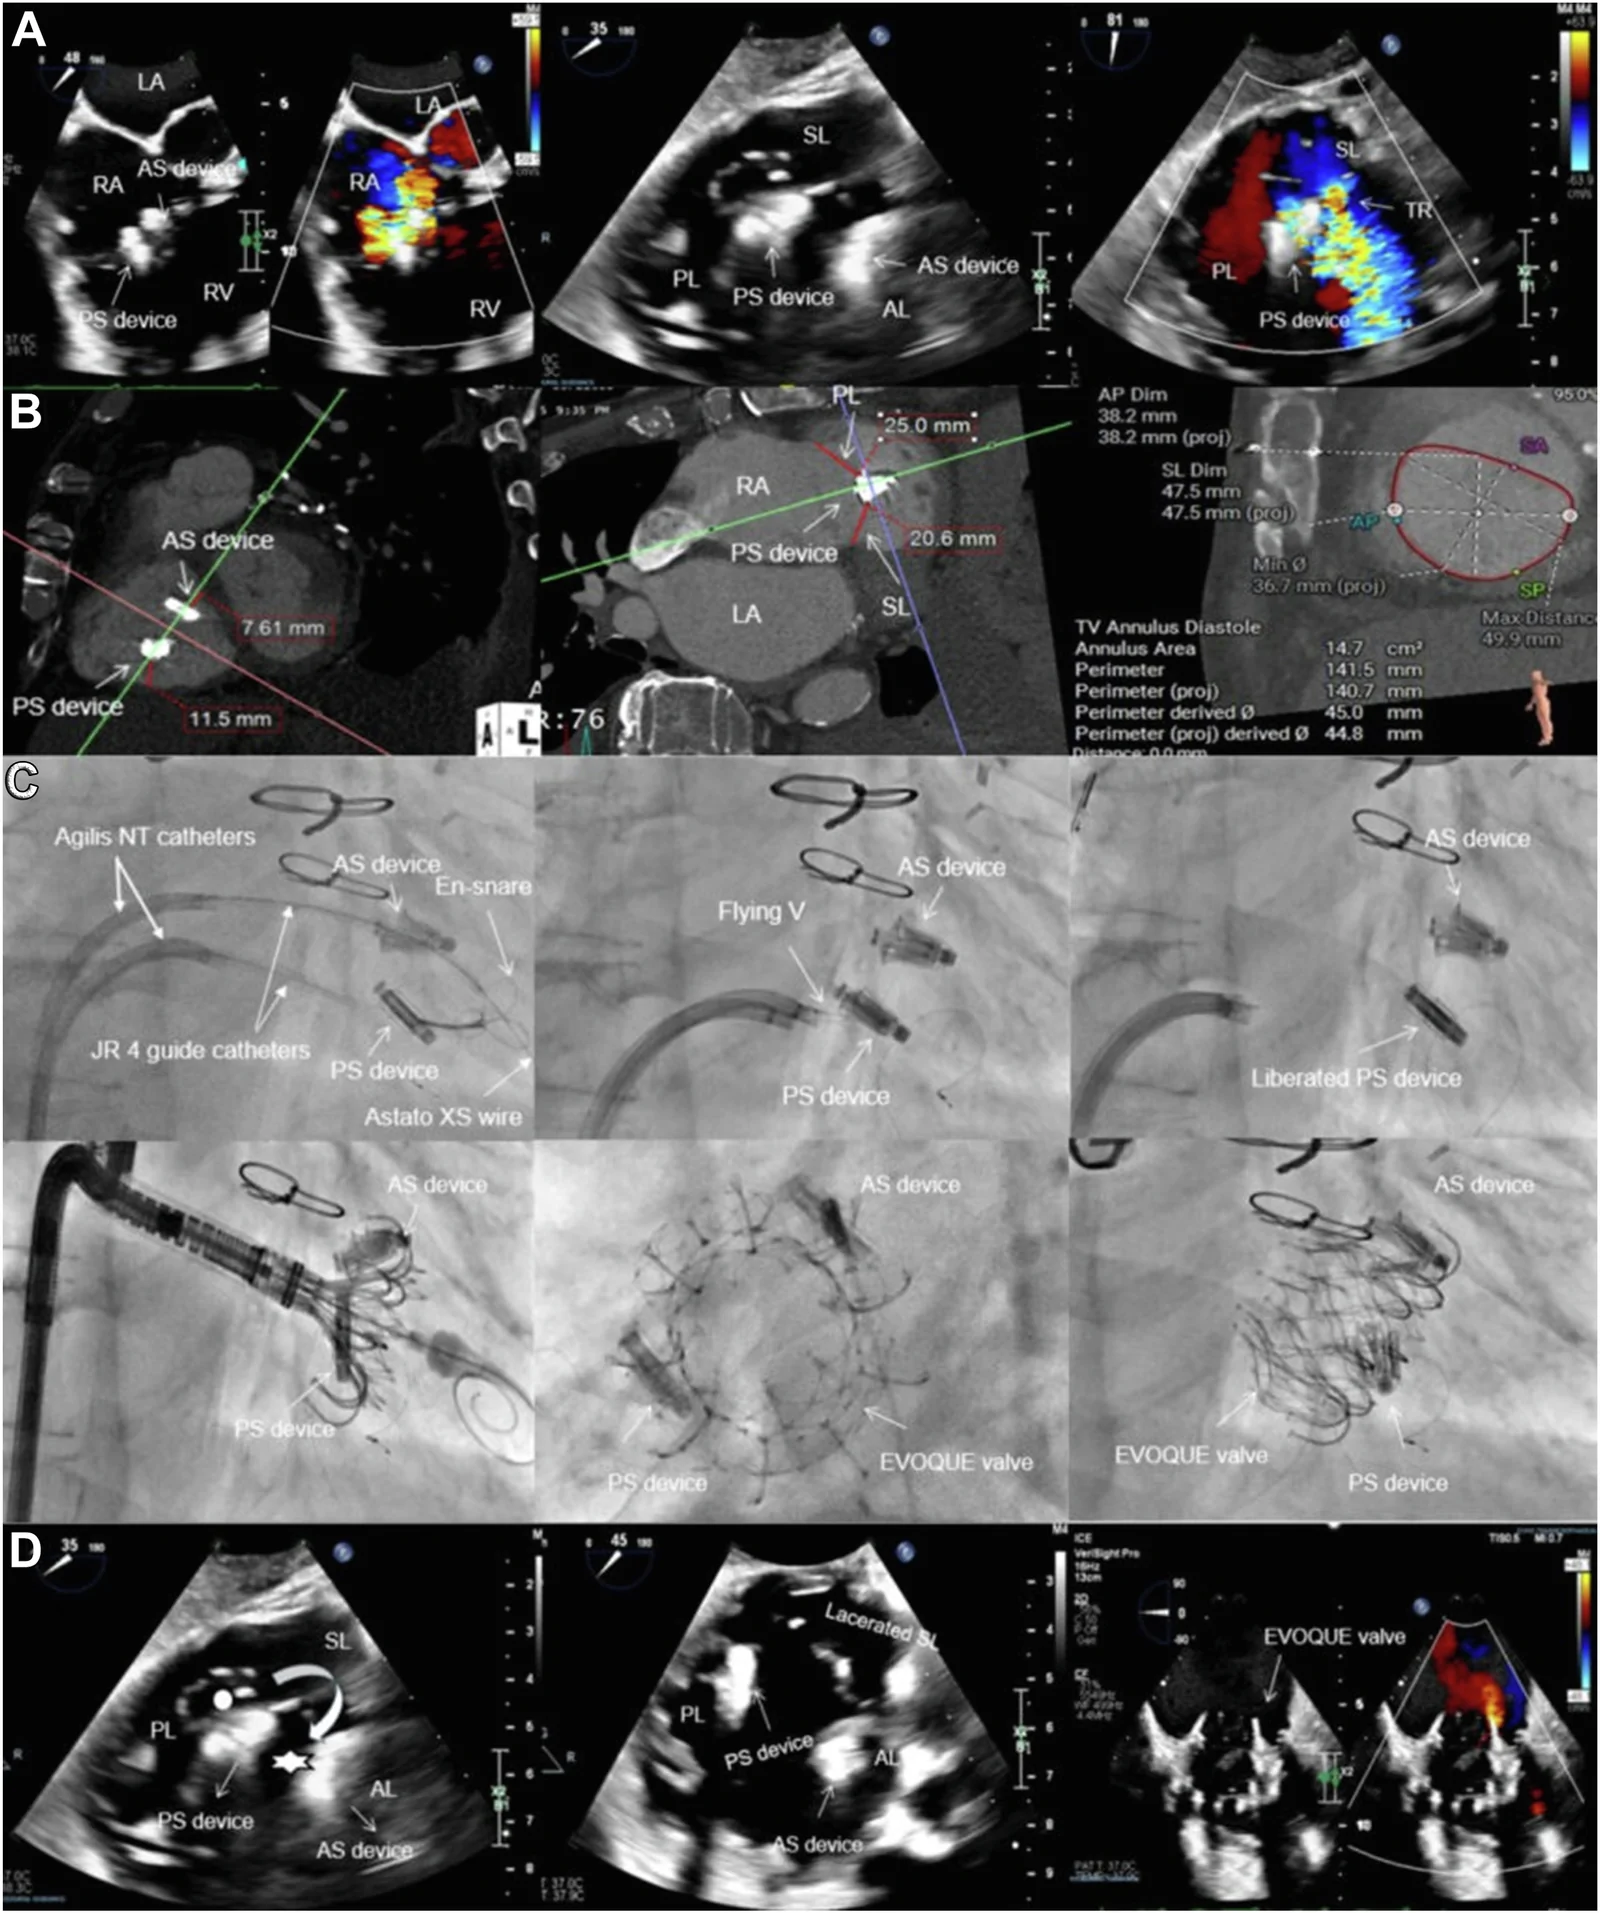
Multimodality imaging of tricuspid TEER device laceration and TTVR. (A) Transesophageal echocardiography demonstrating tricuspid TEER devices and severe TR. Mid-esophageal right ventricular (RV) inflow–outflow view showing anteroseptal (AS) and posteroseptal (PS) TEER devices with color Doppler demonstrating recurrent severe TR. Transgastric short-axis view showing PS device with poor septal leaflet tissue bridge. Severe TR from septal–posterior coaptation gap. (B) Cardiac CT showing device-to-annulus distance, leaflet length, and neo-annular dimensions for TTVR sizing. (C) Fluoroscopy of PS device laceration and TTVR. Astato wire snared with an EN Snare across the PS device. Flying-V configuration around the septal aspect of the PS device. Increased PS device mobility postlaceration. Safari wire in RV and EVOQUE valve deployment. EVOQUE anchors stabilize AS/PS devices. (D) Key transesophageal and intracardiac echocardiographic images of TEER device laceration and TTVR. TEE image showing the planned trajectory of electrocautery laceration (arrow), with the planned orifice for crossing (round and star). Septal leaflet-free PS device. ICE depicting EVOQUE valve with trivial residual TR. ICE, intracardiac echocardiography; TEE, transesophageal echocardiography; TEER, transcatheter edge-to-edge repair; TR, tricuspid regurgitation; TTVR, transcatheter tricuspid valve replacement.
